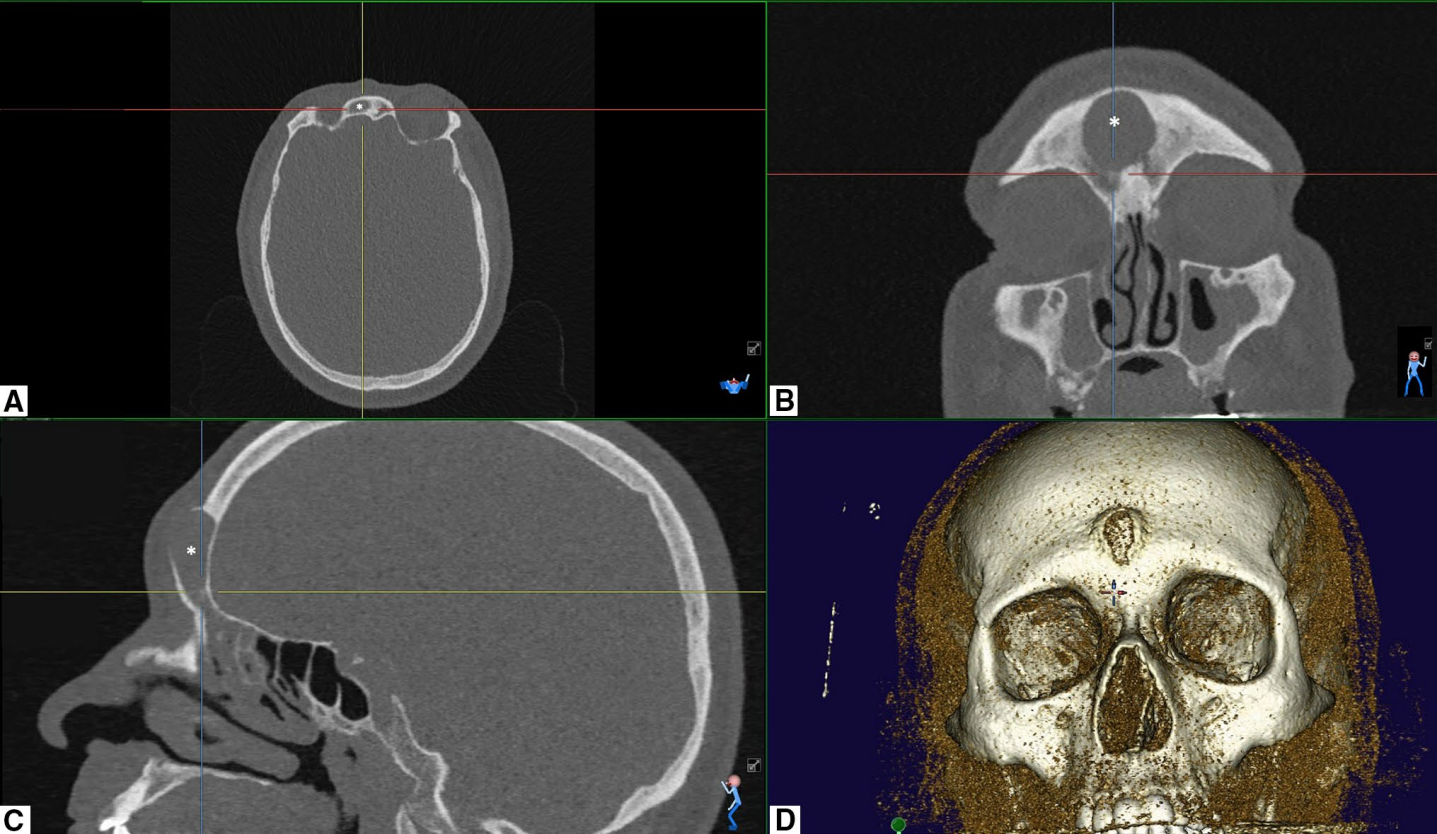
Pre-operative CT scan of the 59-year-old male presenting with Pott’s puffy tumour. Please note the Kuhn type IV cell (*). a Axial, b coronal and c sagittal view. d 3D reconstruction
